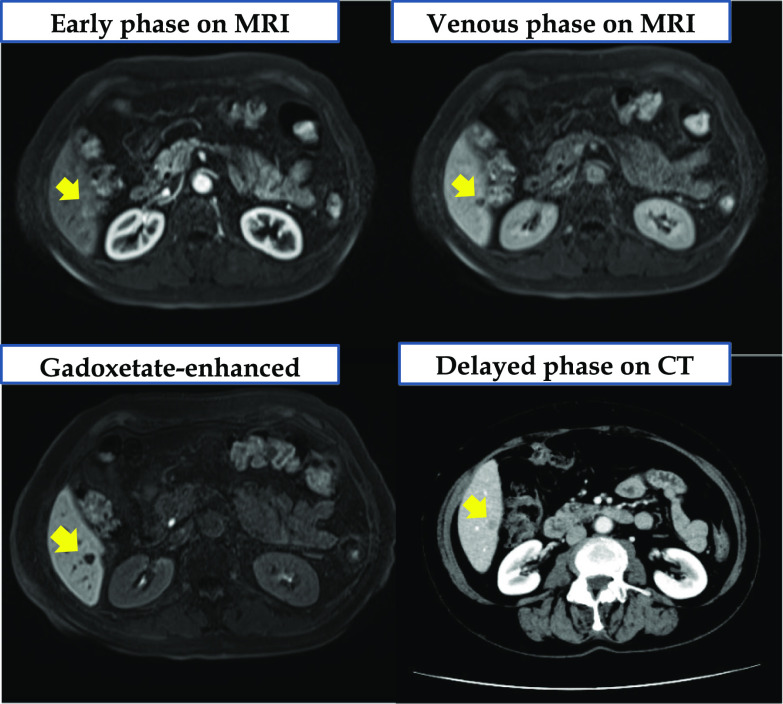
Magnetic resonance image and computed tomography obtained at the 15-year follow-up. On magnetic resonance image, the mass is enhanced in the early phase (arrow) and washed out in the venous phase (arrow). The uptake of gadoxetate sodium is decreased (arrow). On computed tomography, a hypovascular nodule is seen in the delayed phase (arrow)
